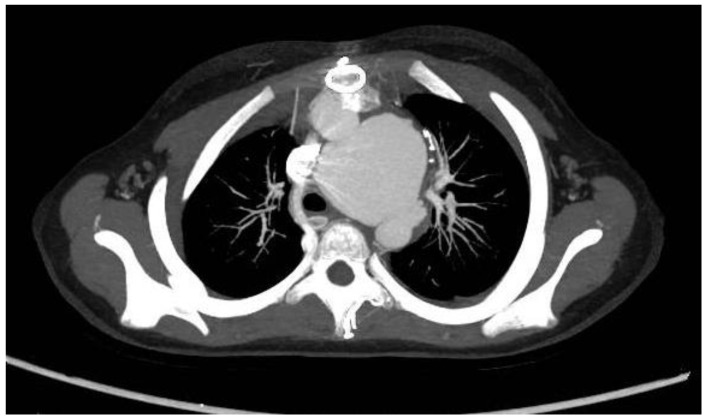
CT angiography of showing pseudoaneurysm of the aortic arch.
